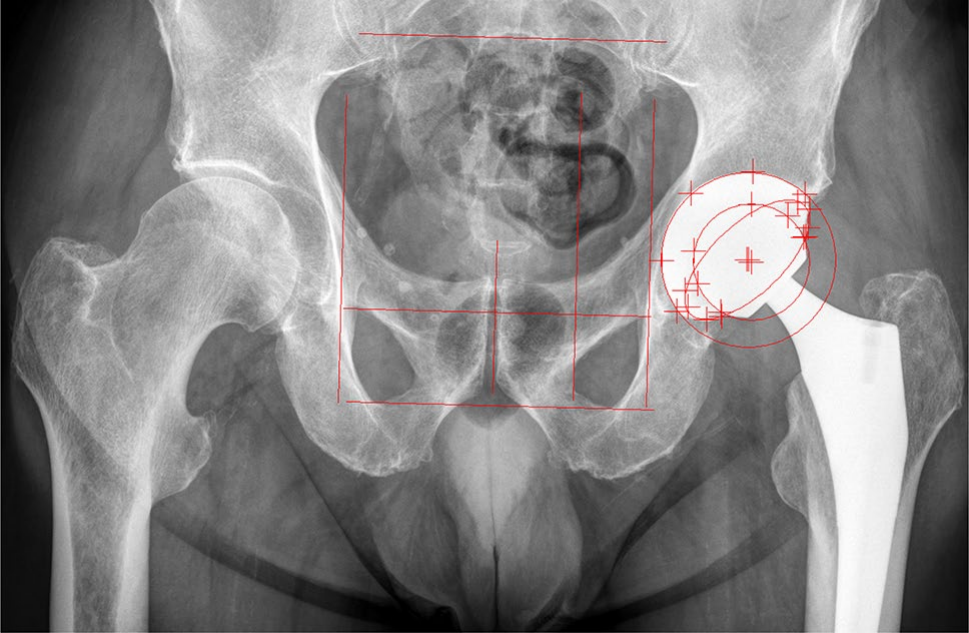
Postoperative anterior–posterior x-ray of a primary total hip arthroplasty with EBRA cup software lines included
